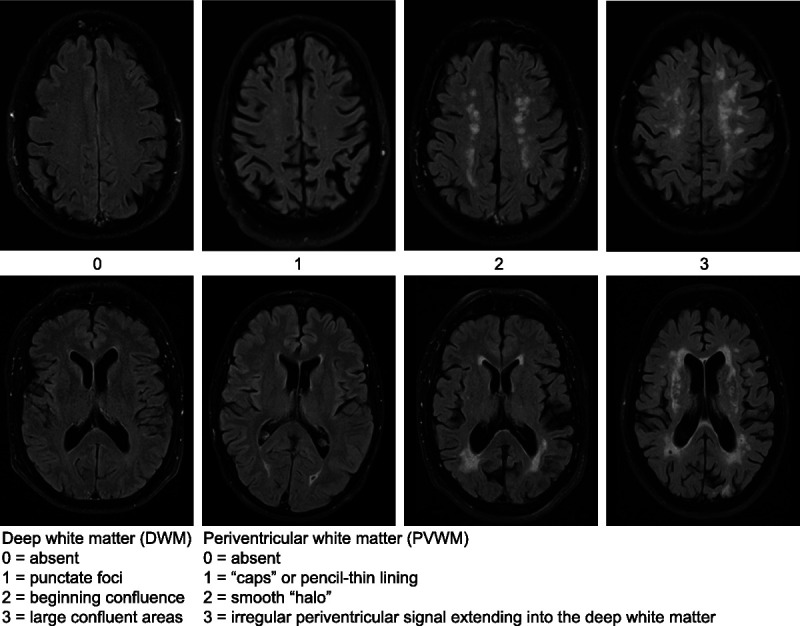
Fazekas scale for MRI imaging. The figure displays hyperintensities in the deep white matter (*upper row*) and periventricular white matter (*lower row*) (24).
